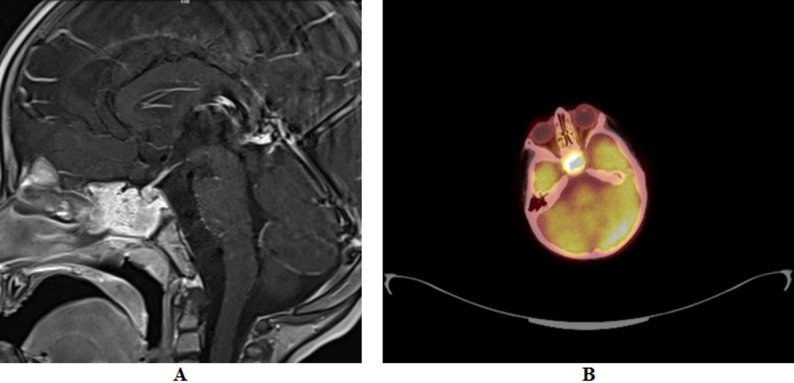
Radiological findings. A Magnetic resonance imaging (MRI) showing hypointense pituitary mass on contrast T1-weighted imaging. B Positron emission tomography (PET) demonstrating hypermetabolic activity with a focus of increased FDG uptake
